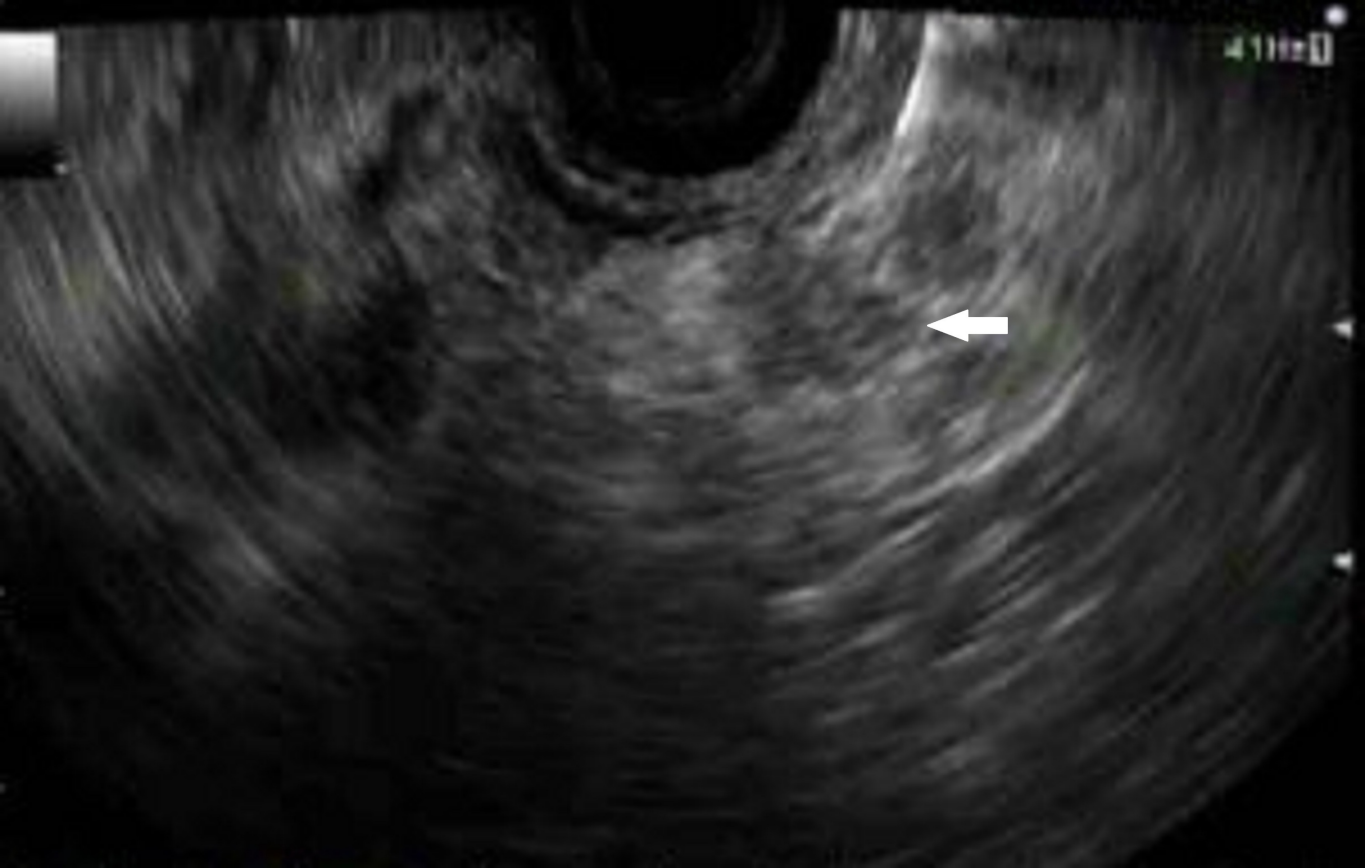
Endoscopic ultrasound image showing a 3.0 x 3.0 cm hypoechoic mass lesion in the pancreatic tail (arrow). The mass was invading into the splenic vasculature. There were multiple malignant-appearing lymph nodes in the peripancreatic area.
